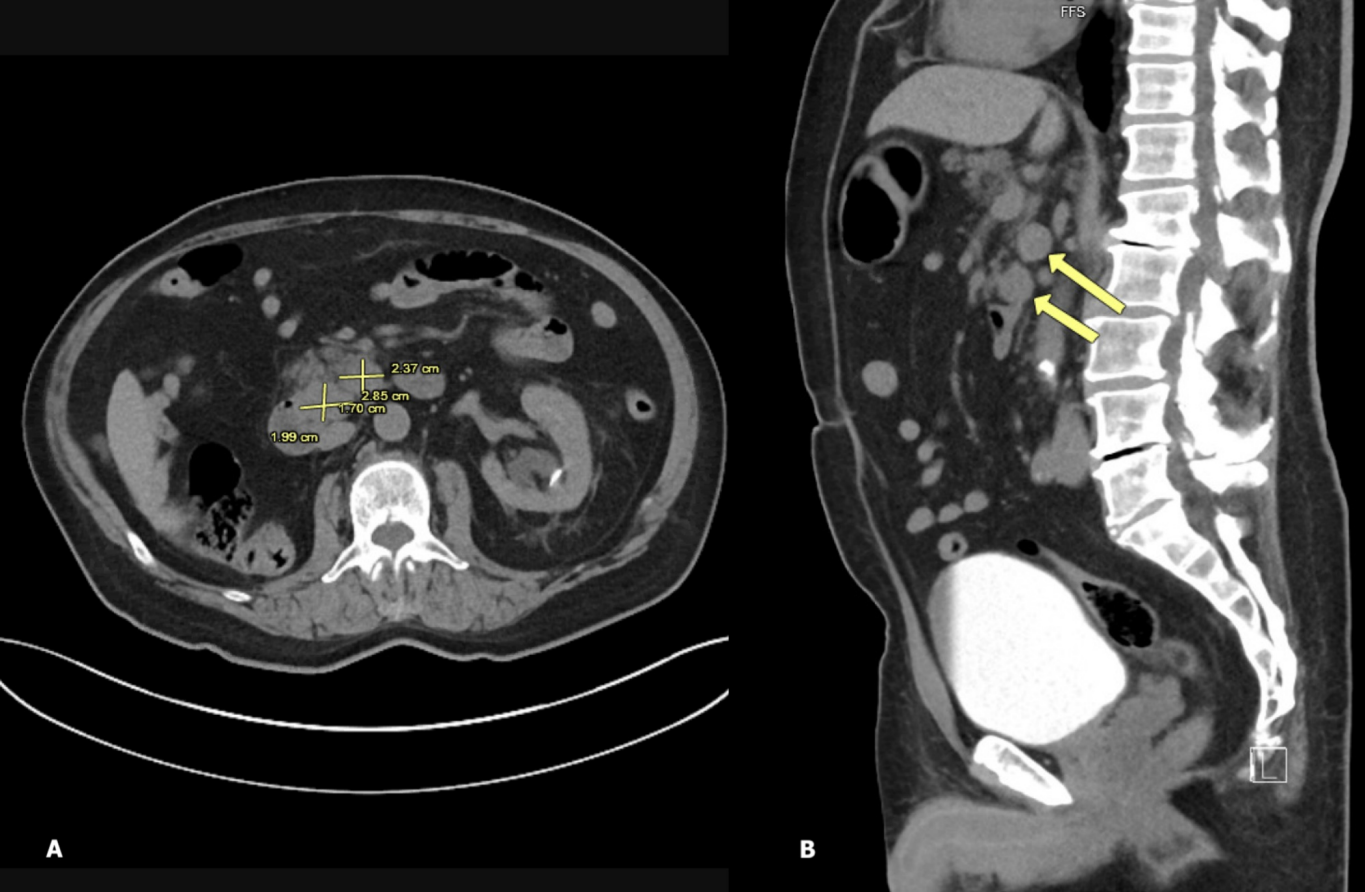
CTAP showing several enlarged LNs in pancreaticoduodenal region – one measuring 2.4 cm x 2.9 cm and another 2.0 cm x 1.7 cm in (A) transverse section (dimensions marked in yellow) and (B) sagittal section (arrows). CTAP, computed tomography abdomen and pelvis; LN, lymph node
